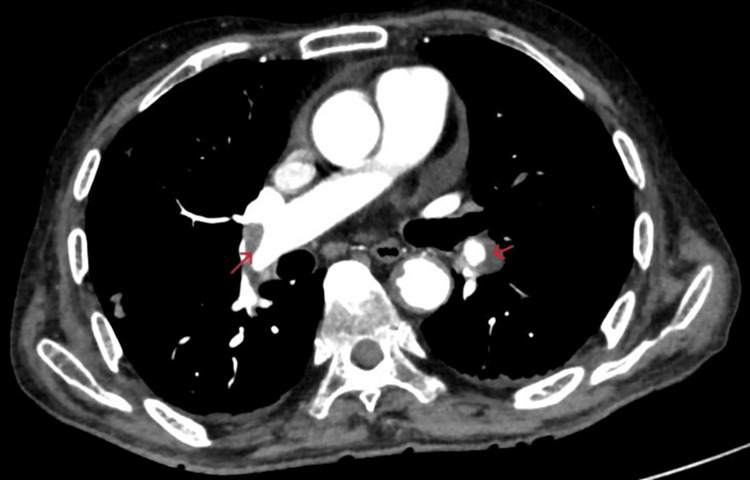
Axial CTPA showing hypodense filling defects (red arrow) in the right interlobar pulmonary artery and segmental branches of the left pulmonary artery. CTPA, computed tomography pulmonary angiography
